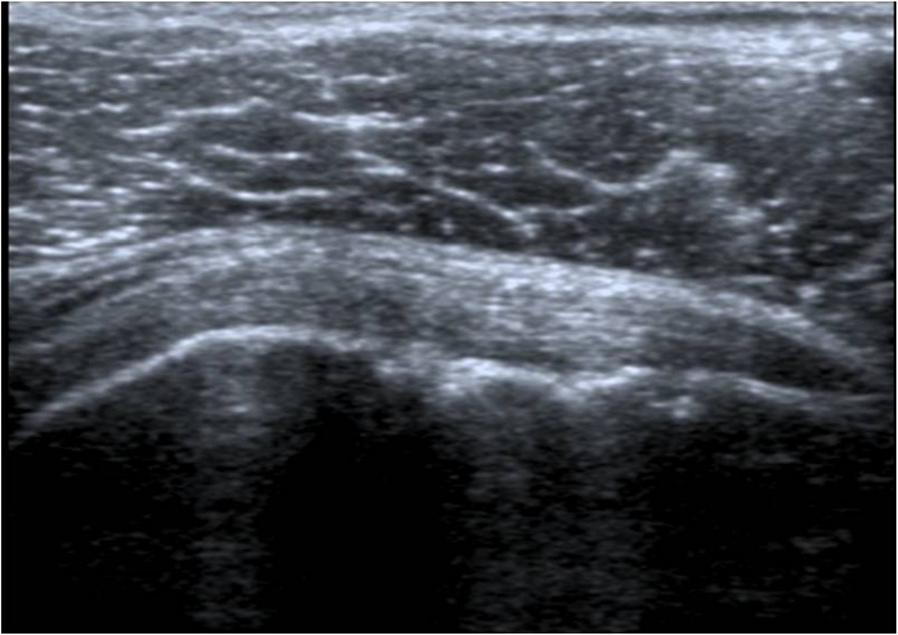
Ultrasound image of subscapularis enthesitis: sagittal B-mode US scaning of subscapularis tendon showing bony erosion with enthesophytes.
